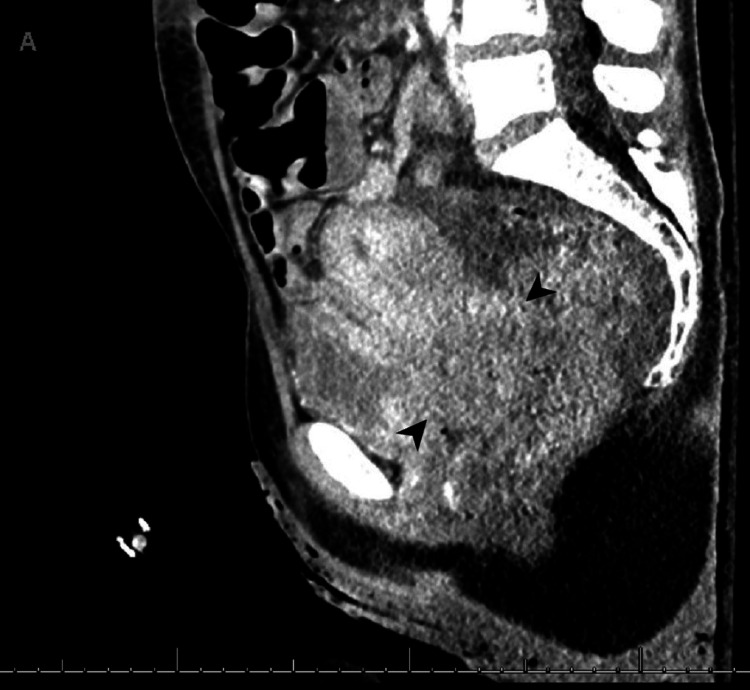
Sagittal view of cervical mass on contrast-enhanced CT scan Black arrowheads bracket the cervical mass.
